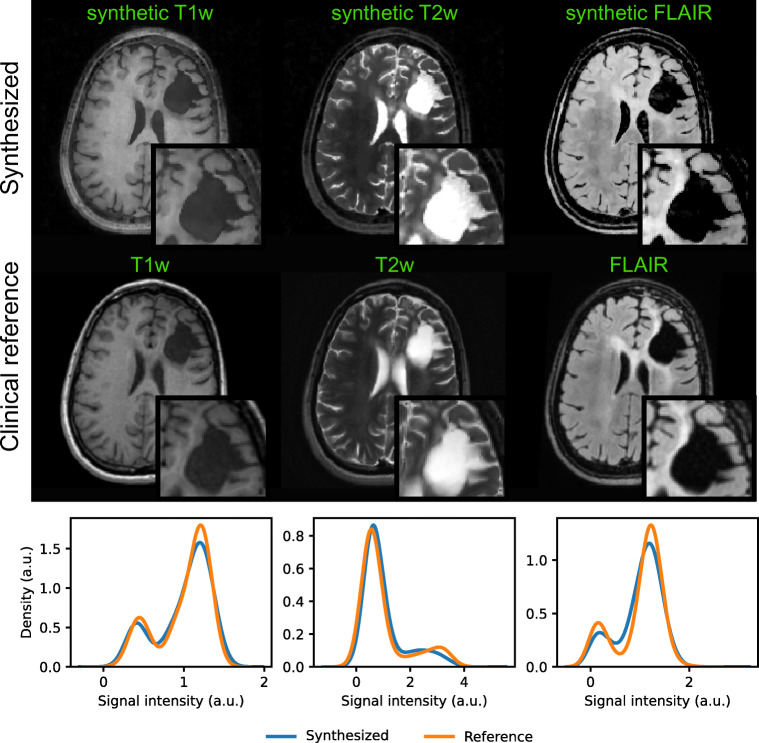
Contrast-weighted image synthesis for a representative patient case. From the T1, T2, and PD maps, we produce clinically relevant, fully 3D qualitative image information with high isotropic resolution and without additional scan time. As seen from the axial views and the histogram-based comparison considering the whole image volumes, synthetic T1-weighted, T2-weighted, and FLAIR MRI contrasts correspond to the clinical reference acquisitions. Corresponding sagittal and coronal views are shown in Fig. 11
